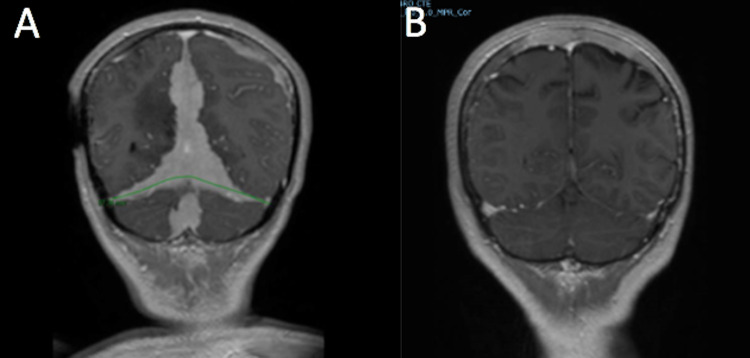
Comparative brain MRI (A) Brain MRI (performed in March 2010): subacute frontotemporal subdural collection, left anterolateral with a temporal predominance, with a maximum thickness of 18 mm with a compressive effect on adjacent parenchyma and asymmetry of the supratentorial ventricular system. (B) Brain MRI (performed in October 2010): persistence of diffuse signal alteration of the bone marrow in the calvarium, with a predominance in parietal regions.
